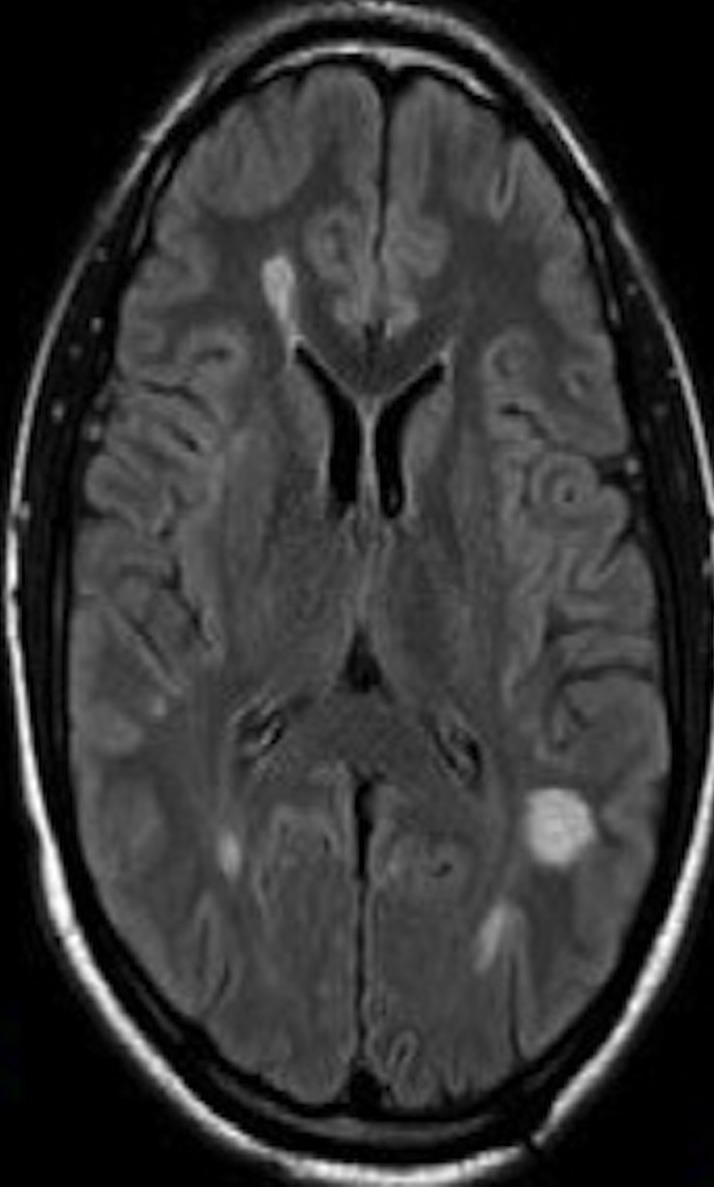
MRI after antimicrobial therapy shows decreased size of the lesions. Decreased white lesions seen in MRI
